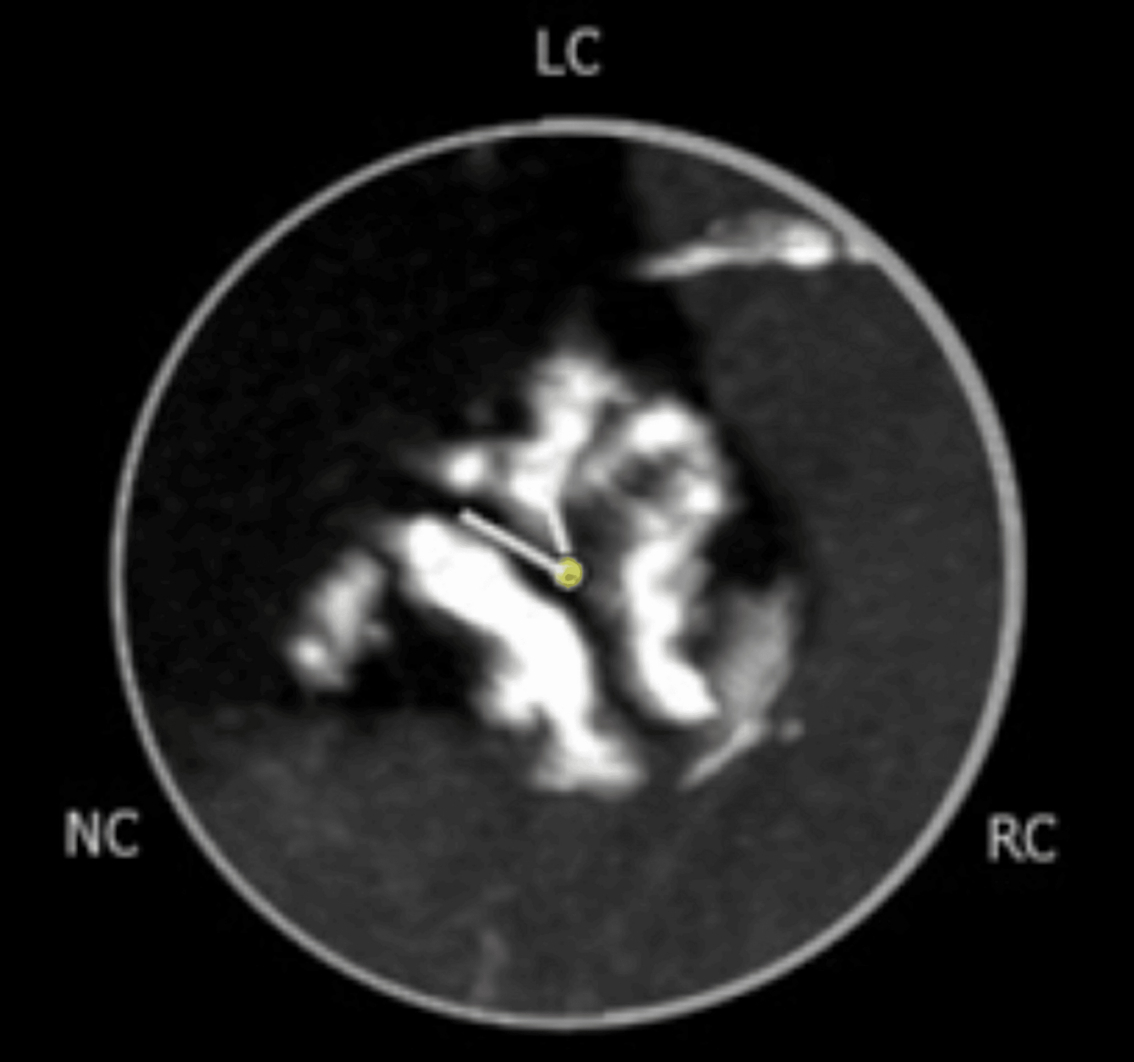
Cardiac CT image of the heavily calcified aortic valve before TAVR was performed. TAVR: transcatheter aortic valve replacement, LC: left cusp, NC: non-coronary cusp, RC: right cusp
